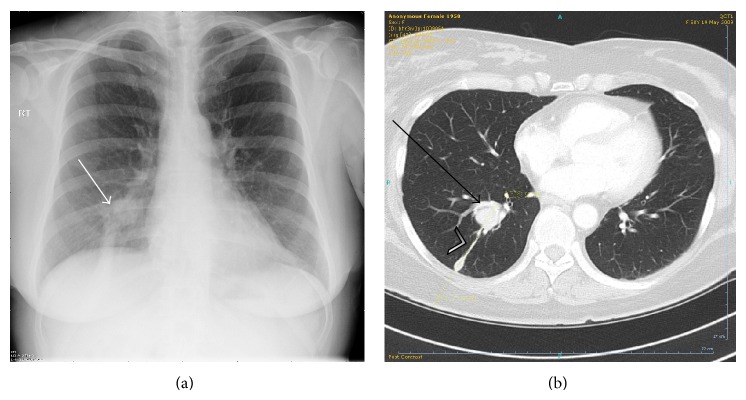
(a) Chest X-ray showed a 2 cm round opacity (white arrow) over the lower end of the right hilum with an inferiorly extending band shadow. (b) Transverse section chest contrast-enhanced computed tomography confirmed a 26 mm by 23 mm irregularly round lesion (black arrow) located at the bifurcation of the posterior segmental division of the lower lobe bronchus. There is further extension posteroinferiorly of the irregular soft tissue (arrowhead) contiguous from the lesion towards the costophrenic angle and diaphragm with pleural thickening.
